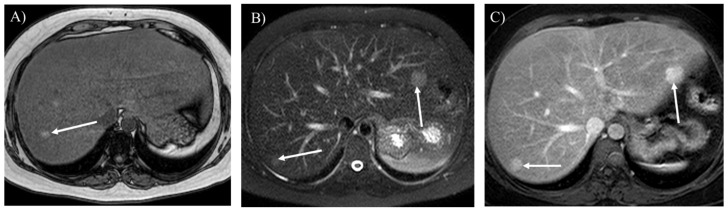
Opposed-phase T1-weighted imaging (A) demonstrates hepatic steatosis and hepatic adenoma in a 31-year-old woman with glycogen storage disease type Ia (arrow). T2-weighted (B) and contrast-enhanced T1-weighted (C) imaging show hepatic adenomatosis (arrows).
